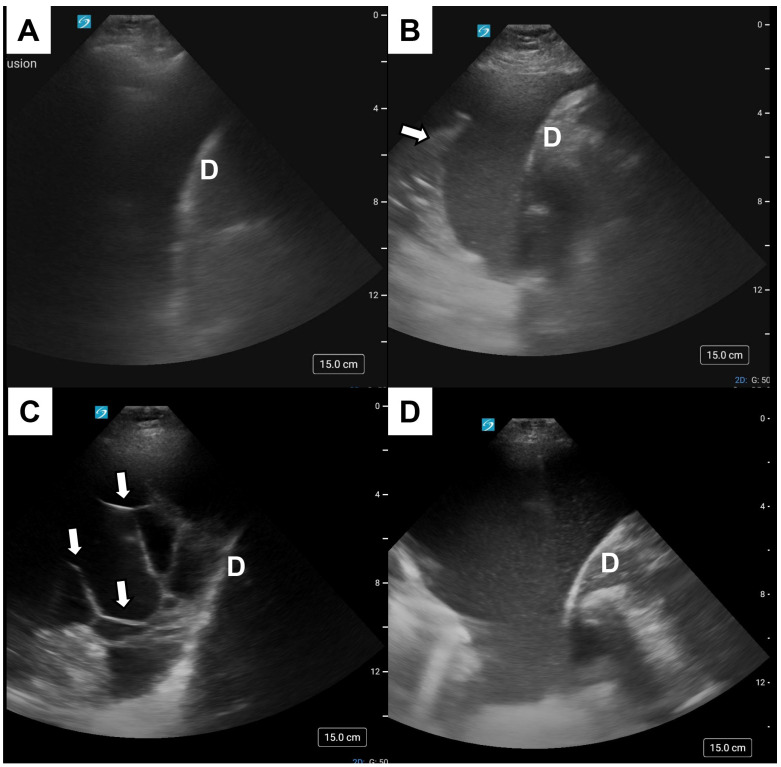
Pleural ultrasound characteristics. Ultrasound of the pleural space is categorized as simple (A) and complex (B–D). Categories of complex ultrasound include complex non-septated (B) noted with atelectatic lung (white arrow), complex-septated (C) with loculations (white arrows), and homogenously echogenic (D).
